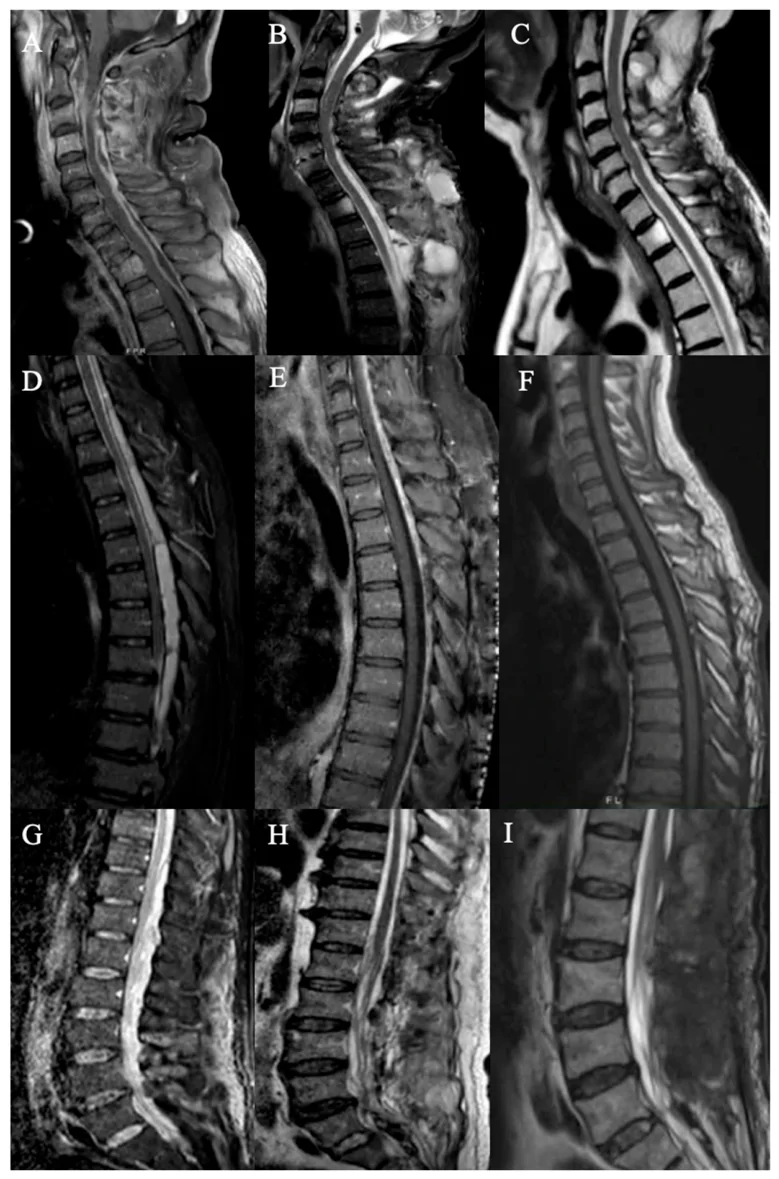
Preoperative, early postoperative, and 6-month postoperative sagittal MRI scan of 3 representative cases of spinal epidural abscess due to spondylodiscitis, successfully treated with emi-laminectomy. The first case (panels (A–C)) is a 68-year-old male with cervical (C4–C6) and thoracic (T3–T5) epidural abscess. The second case (panels (D–F)) is a 39-year-old male with thoracic (T5–T10) epidural abscess. The third case (panels (G–I)) is a 73-year-old male with thoracolumbar (T9–S1) epidural abscess. Across all 3 cases, early postoperative MRI demonstrated restoration of canal patency, and 6-month imaging showed maintenance of decompression with resolution of the compressive component despite residual structural changes typical of healed infection.
